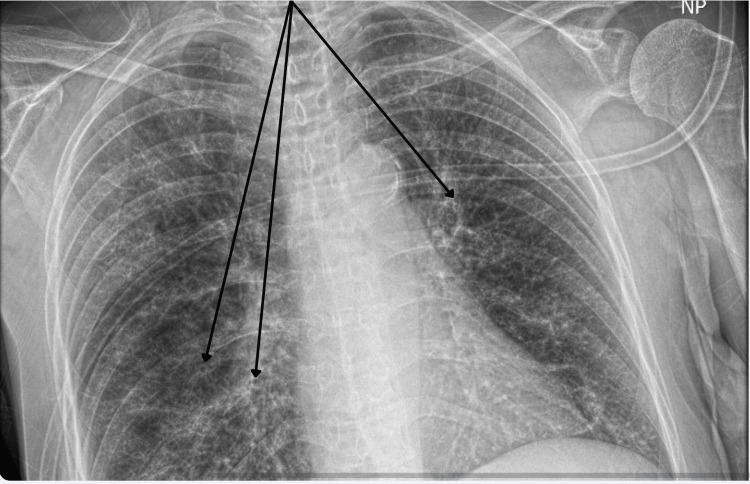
Initial Chest Radiograph The arrows demonstrate examples of the described diffuse bronchial wall thickening.
